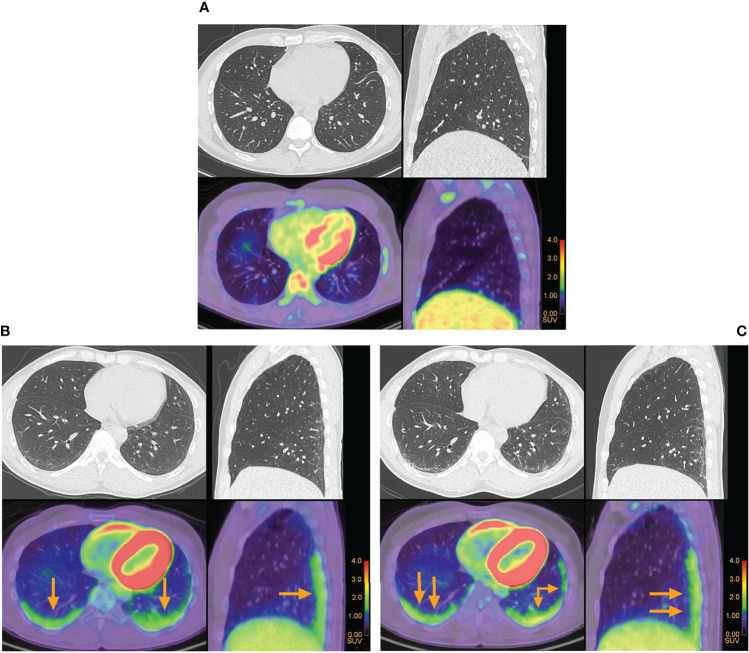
Pulmonary ^18^F-FDG positron emission tomography and high resolution computed tomography in patients with early severe diffuse cutaneous systemic sclerosis. (A) No visual uptake of ^18^F-FDG was observed in a patient with systemic sclerosis without interstitial lung disease. (B) Increased uptake of ^18^F-FDG in the lungs of a patient with systemic sclerosis associated interstitial lung disease is indicated by the arrows. (C) Repeated ^18^F-FDG positron emission tomography in the same patient, who had severe progression of interstitial lung disease after 3 months of cyclophosphamide pulse treatment. Higher uptake of ^18^F-FDG was seen at the follow-up scan when compared to baseline.
